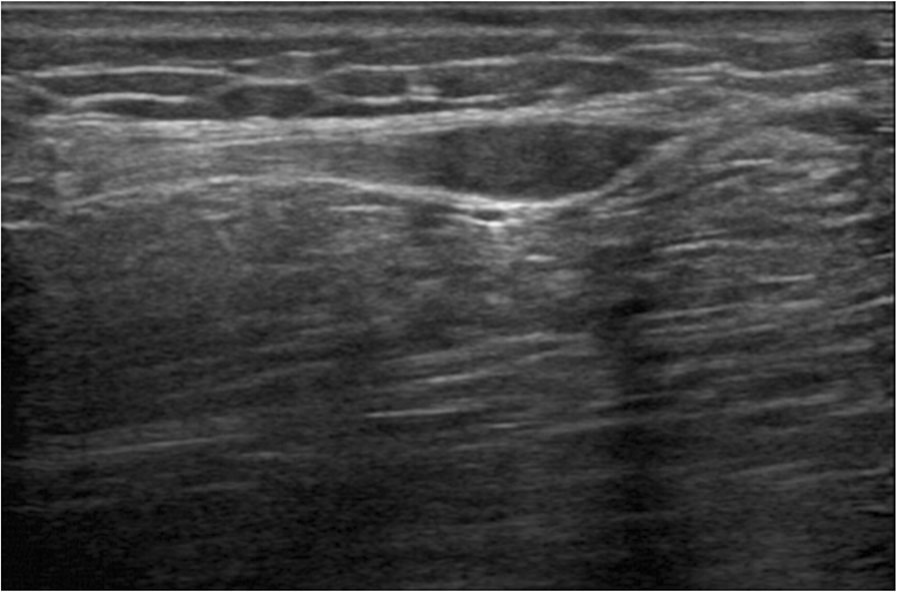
B ultrasound: a 12.0 × 5.0 mm fusiform hypoechoic nodule can be found between the right calf fat depth layer and muscle layer, with clear boundary and both ends connected to the fiber ribbon cord-like structures
